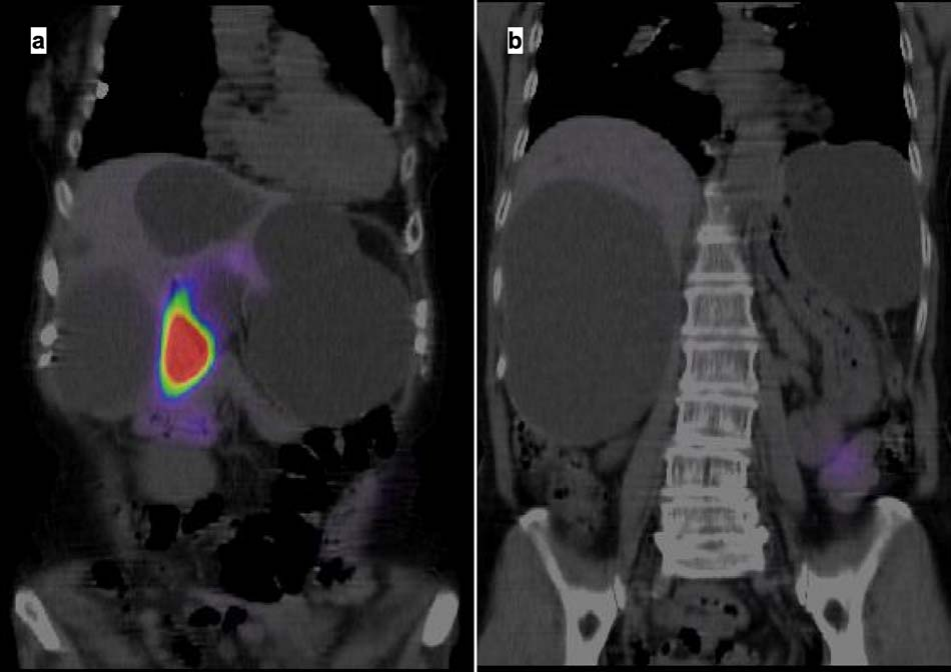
Hepatobiliary scintigraphy with CT highlighting only the gallbladder, with no biliary communication with the liver cysts.
